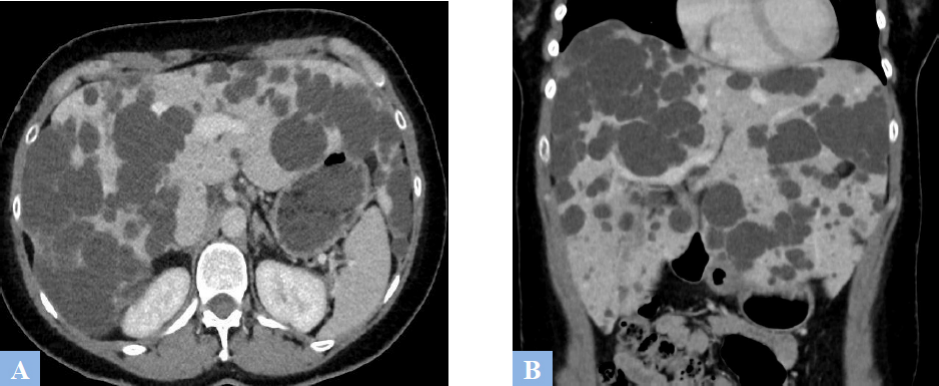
Uncomplicated hepatic polycystic disease. A. CT image in the axial plane at the portal phase of enhancement. Multiple well-defined hypodense hepatic cysts involving all hepatic segments. B. Same CT scan, coronal reconstruction. Liver enlargement due to the multiple biliary cysts.
